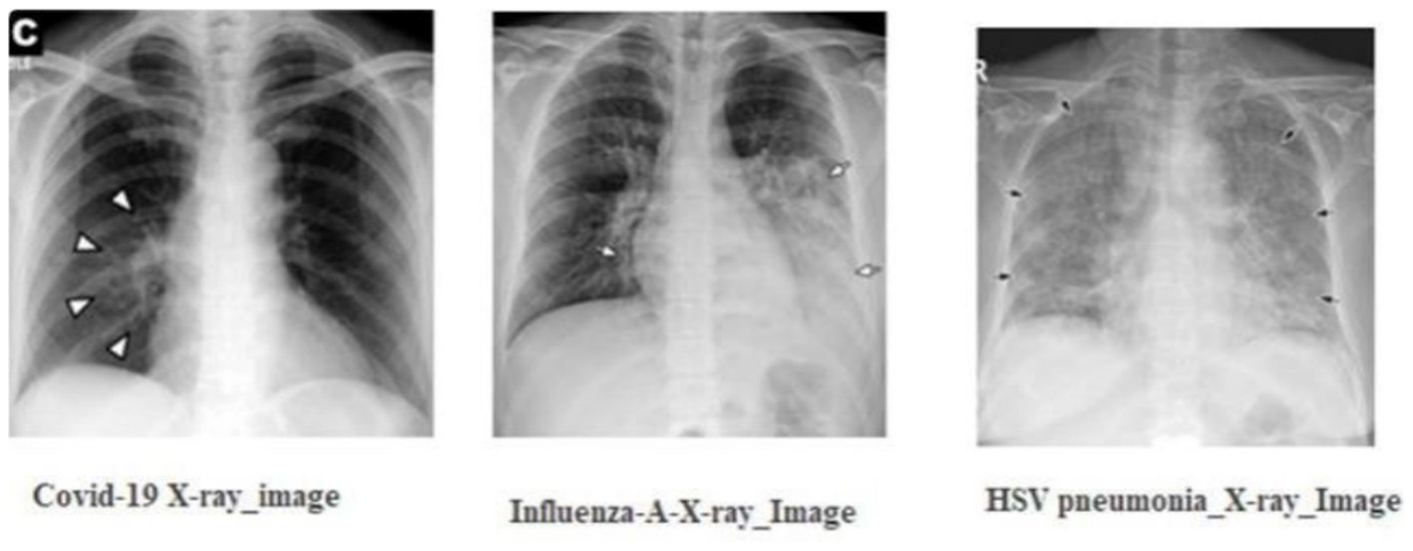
The radiographic characteristics indicative of COVID-19, showing bilateral and lower-zone dominant ground-glass opacities (GGOs) along with consolidations.
